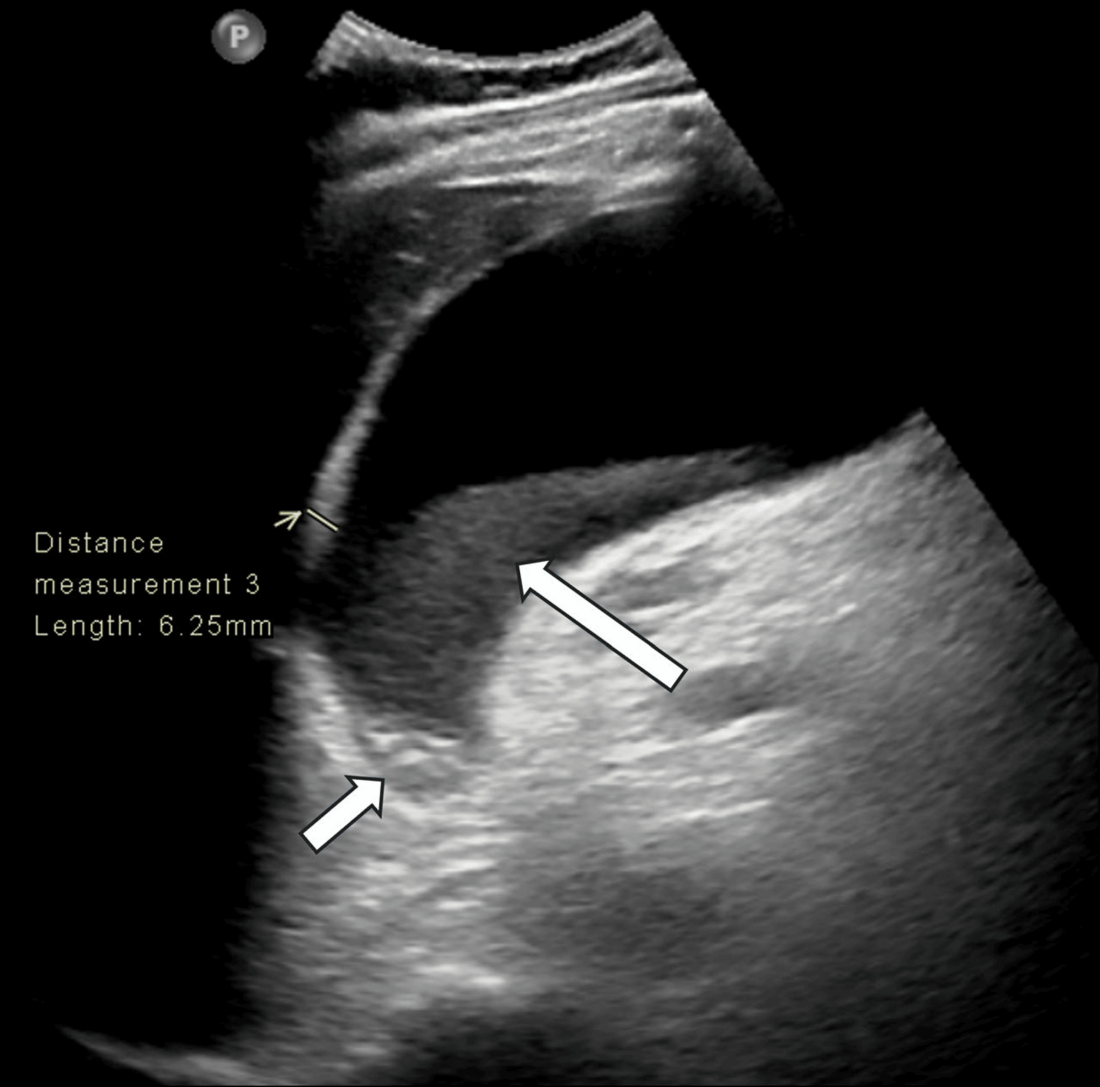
Abdominal ultrasound Abdominal ultrasound showing an over-distended gallbladder (11 cm in the long axis) with irregular wall thickening (up to 6 mm), sludge (long white arrow), calculi (short white arrow) impacted at the gallbladder neck, and positive sonographic Murphy sign. There was no associated biliary dilatation or stones. The appearance is consistent with noncomplicated acute calculous cholecystitis.
